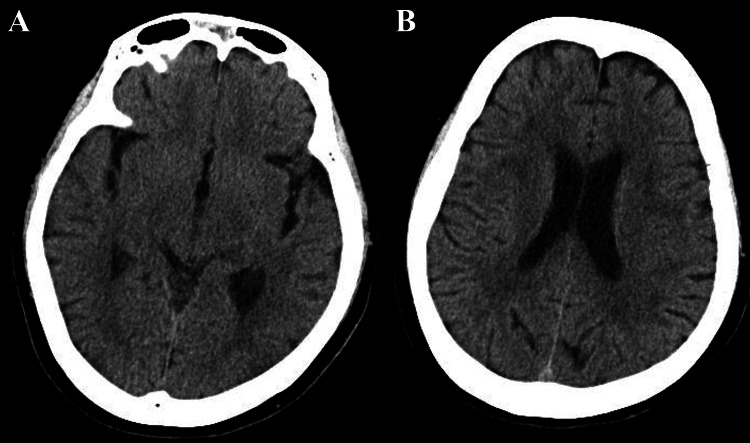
Head computed tomography (CT) revealing no hemorrhage or calcification (A, B).
